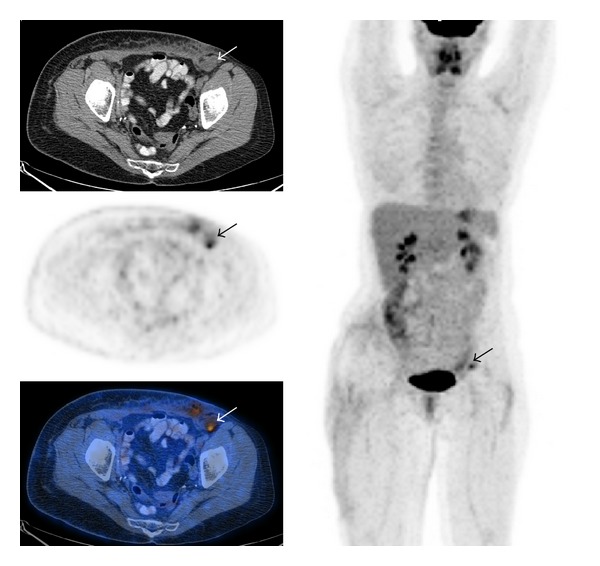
True positive that changed management; in 45-yea-old patient with a recently resected left lower abdominal wall angiosarcoma, PET/CT demonstrated uptake in the left inguinal region (arrow). This was subsequently biopsied and proven to be metastatic angiosarcoma.
